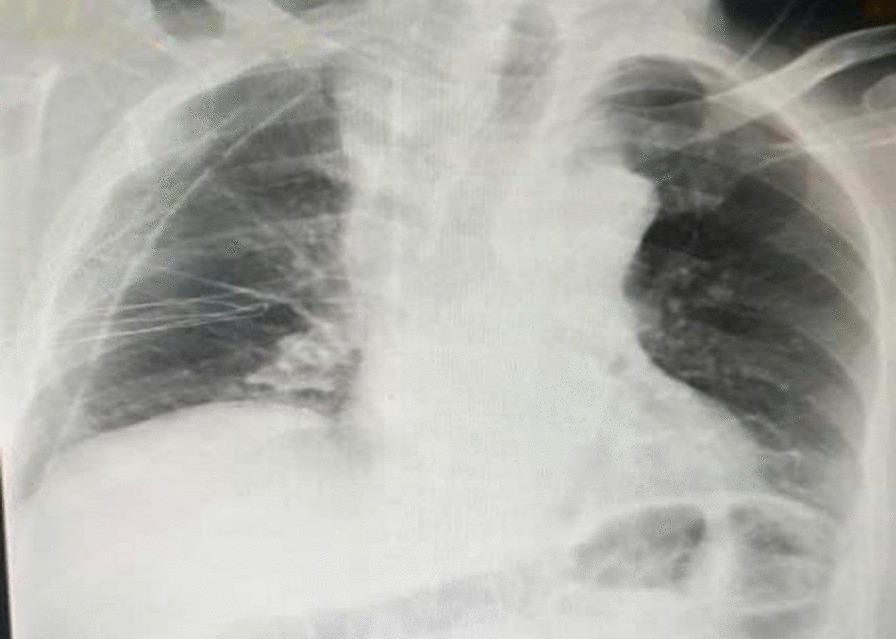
Follow up chest X-Ray demonstrating clear lungs and a central mediastinum of normal size
